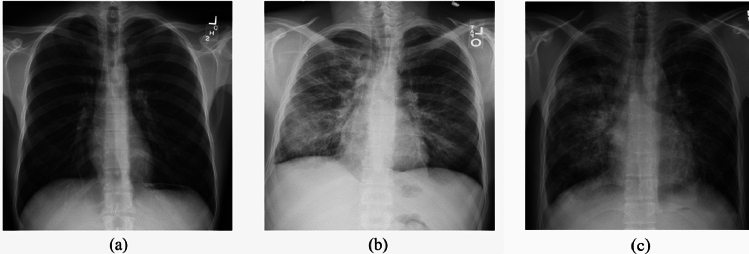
Sample images from the ChestX-ray14 dataset: a Normal CXR, b Pneumonia case, and c Infiltration case. The pathologies in (b, c) can be distinguished from the normal case in (a) by the presence of abnormal opacities in the pulmonary lobes. However, b, c are not easily distinguishable from each other due to similar radiographic appearances
